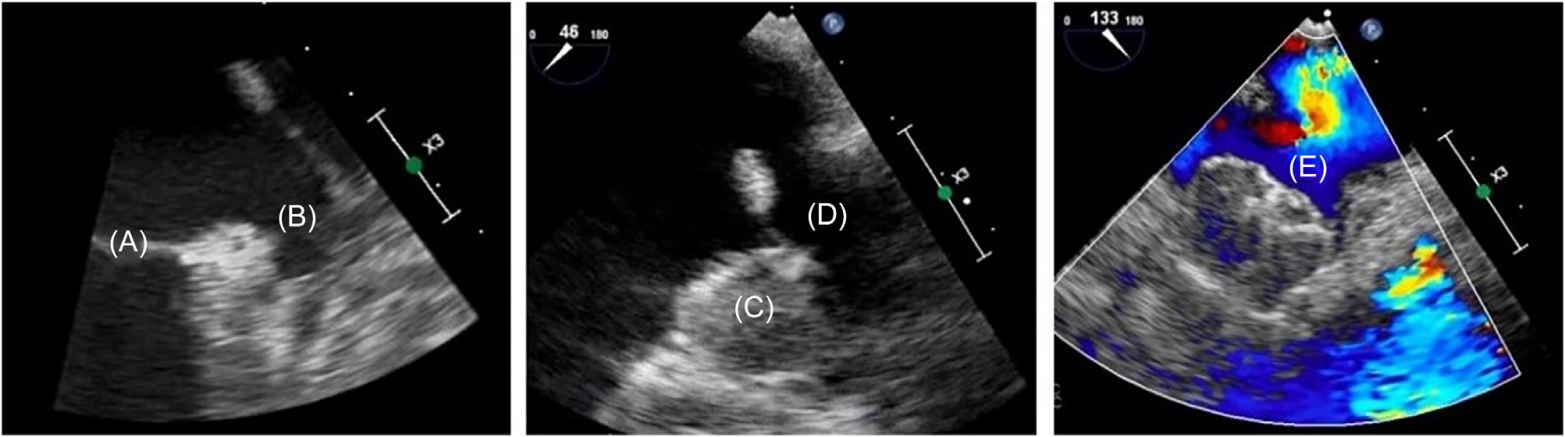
Transesophageal echocardiography: (A) Posterior leaflet of the mitral valve. (B) Left atrial appendage pre‐Watchman placement. (C) Left atrial appendage now with Watchman occluding the os. (D) Left upper pulmonary vein. (E) Color Doppler demonstrating no residual flow around the Watchman device.
